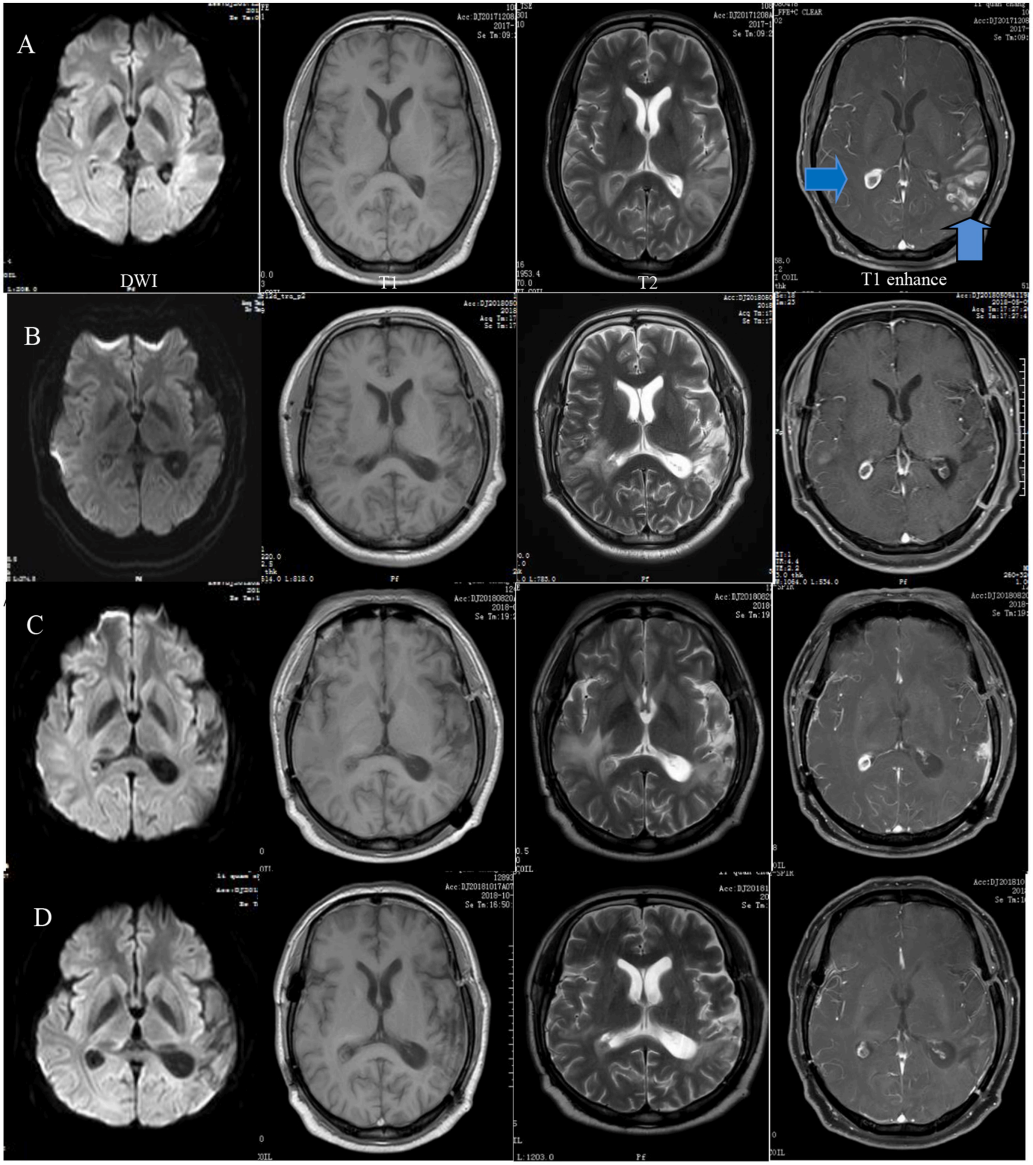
Preoperative MRI showing a wide range of long T1 and T2 signals in the left temporal, occipital, and insular lobes and diffusion-weighted imaging (DWI) demonstrating restricted diffusion in the same area as well as a right quasi-circular ring-enhancement after gadolinium administration (A). A follow-up MRI 5 months after surgery demonstrated basically stable changes (B). Eight months later, the extended range of edema became more apparent. Enhancement of the frontotemporal lobe was visible at the surgical site (C). The range of edema and degree of enhancement were obviously reduced after 1 month of therapy (D).
